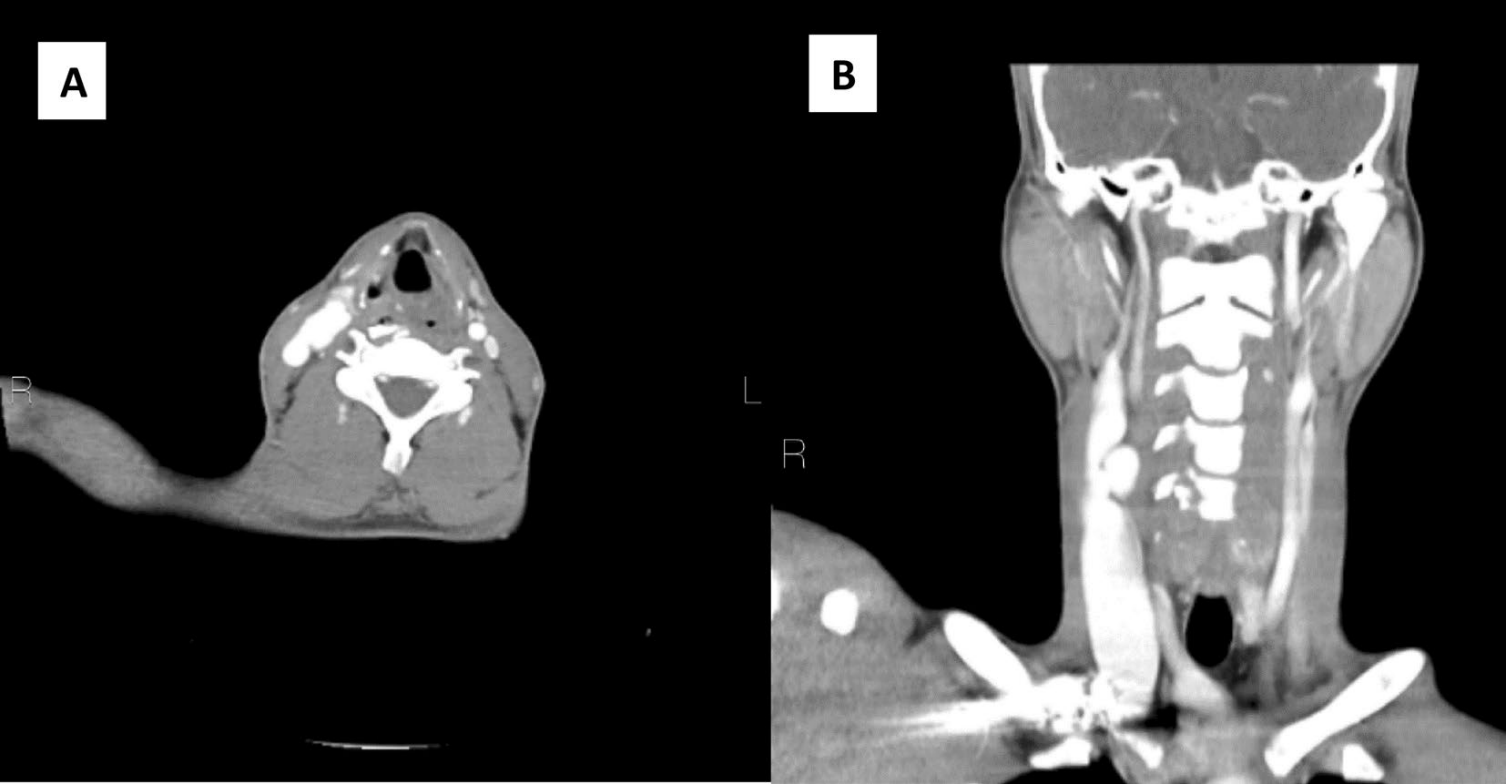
Spiral CT‐Scan of neck with IV Contrast (A: Axial View—B: Coronal View). Aneurysmal dilation of the distal CCA (15*14 mm) with fistula to the right Jugular Vein (AVF).
